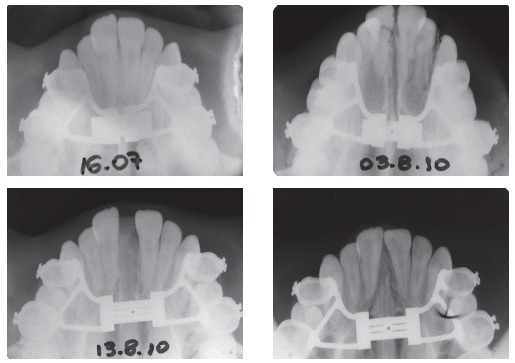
Pre-RME and post-RME occlusal radiographs.
